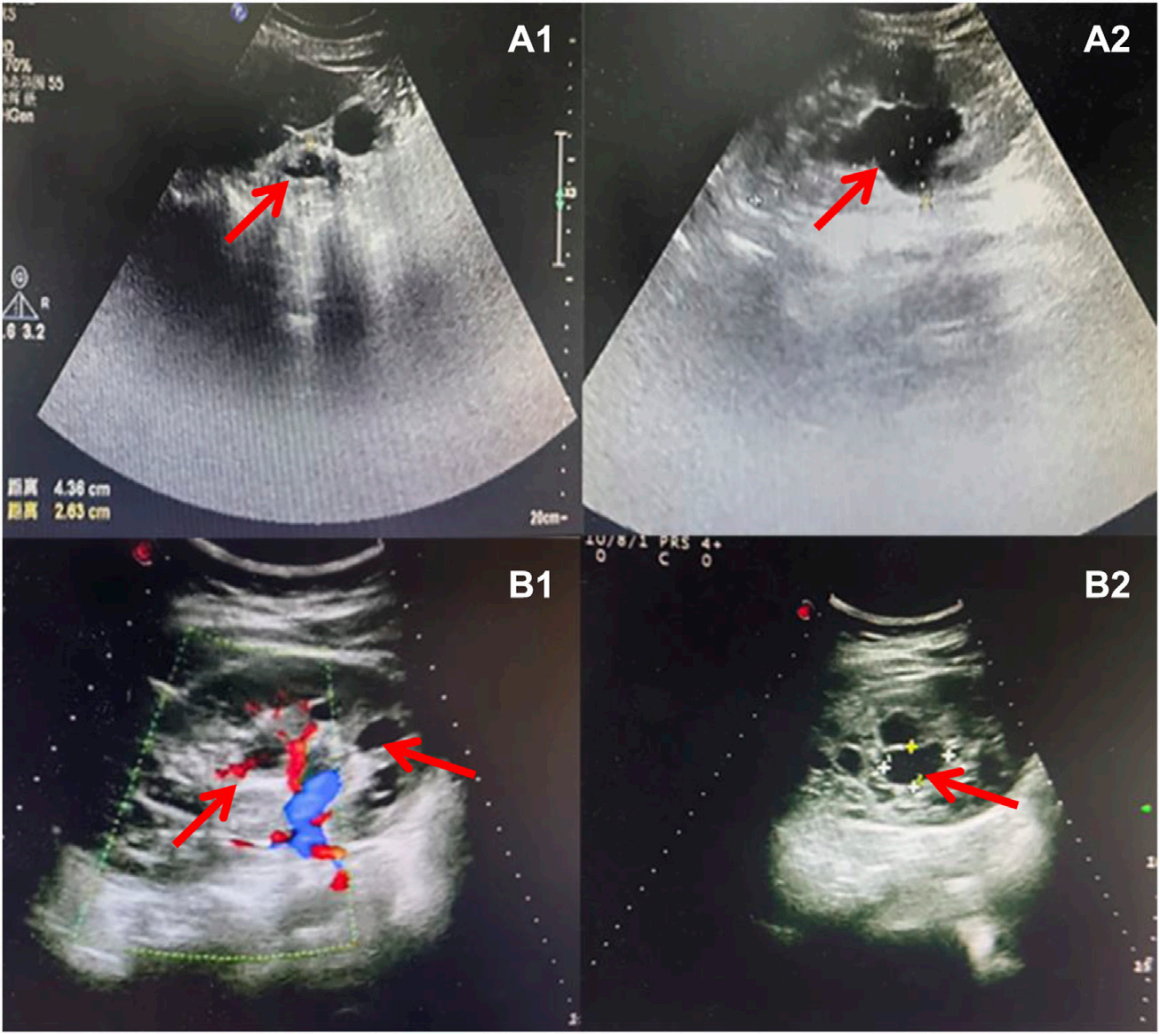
Sonographic characteristics of renal anomalies of the father (case 2) and mother (case 3) of affected fetuses. Sonographic images indicated that the father of case 2 presented with bilateral multiple renal cysts (A1), with the biggest kidney cyst measuring 2.9 cm by 2.6 cm (A2). Sonographic images revealed that the mother of case 3 had right renal atrophy with renal cyst (B1) and left hydronephrosis (B2).
